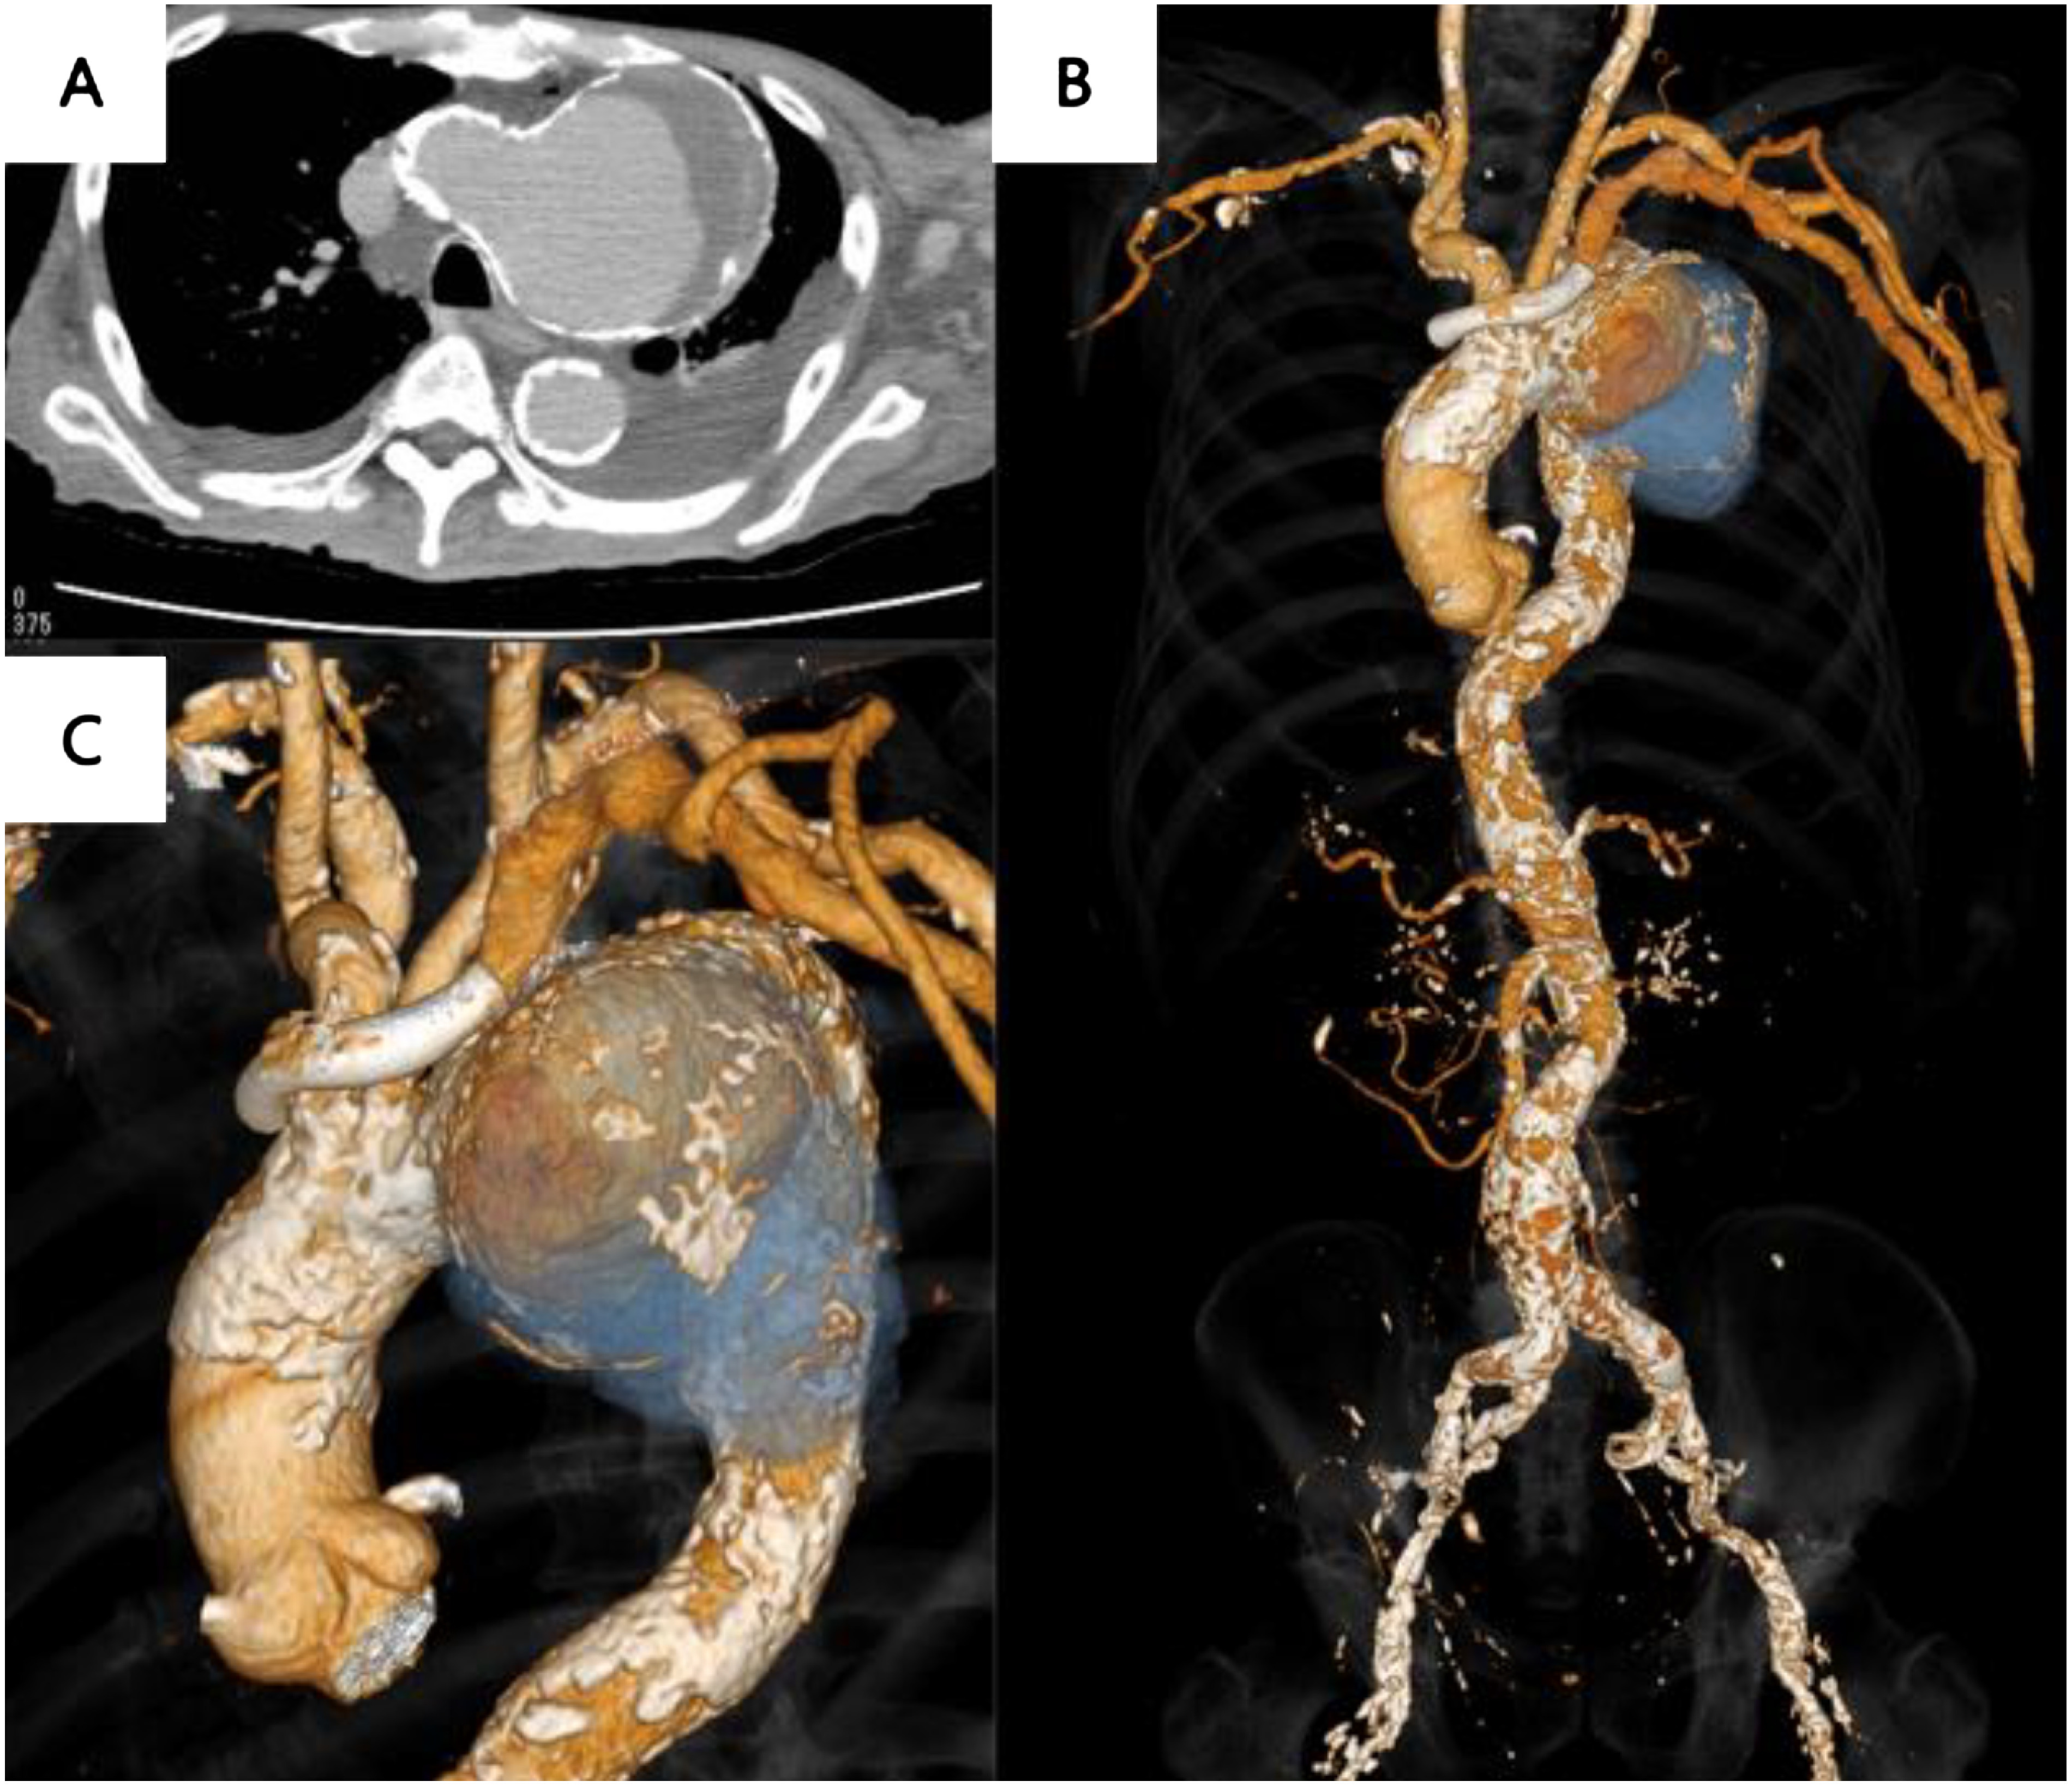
Fig. 1 (A) (B) Chest computed tomography (CT) scan reveals an aortic arch aneurysm with extremely severe aortoiliac calcification. (C) A bare-metal stent was inserted into the left brachiocephalic vein at another hospital because of arteriovenous fistula stenosis.
